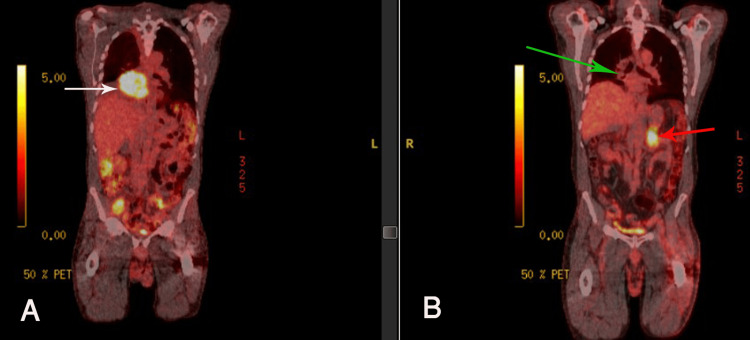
NM PET-CT before (A) and after (B) treatment with carboplatin, paclitaxel, and atezolizumab. Image (A) is remarkable for FDG-avid right hilar mass (white arrow) measuring 8.5 x 6.8 x 6.3 cm with SUV max of 11.3. Image (B) shows decreasing patchy consolidation in the right infrahilar region (green arrow) without significant focal uptake, SUV max of 1.9, previously 2.5, and new FDG avid left adrenal mass (red arrow) suspicious for metastasis. NM PET-CT: nuclear medicine positron emission tomography-computed tomography; FDG: F-18 fluorodeoxyglucose
